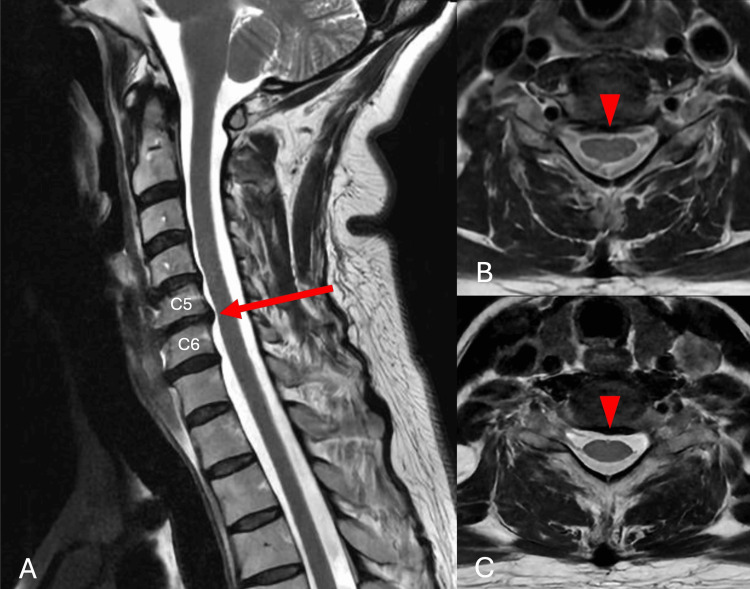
Preoperative cervical spine T2-weighted MRI. (A) Sagittal image showing disc bulging at C5-C6 (red arrow) and C6-C7 with an inferior C5 osteophyte. Axial images at (B) C5-C6 and (C) C6-C7 demonstrating central disc bulging (arrowheads) and without cord compression or foraminal stenosis.
